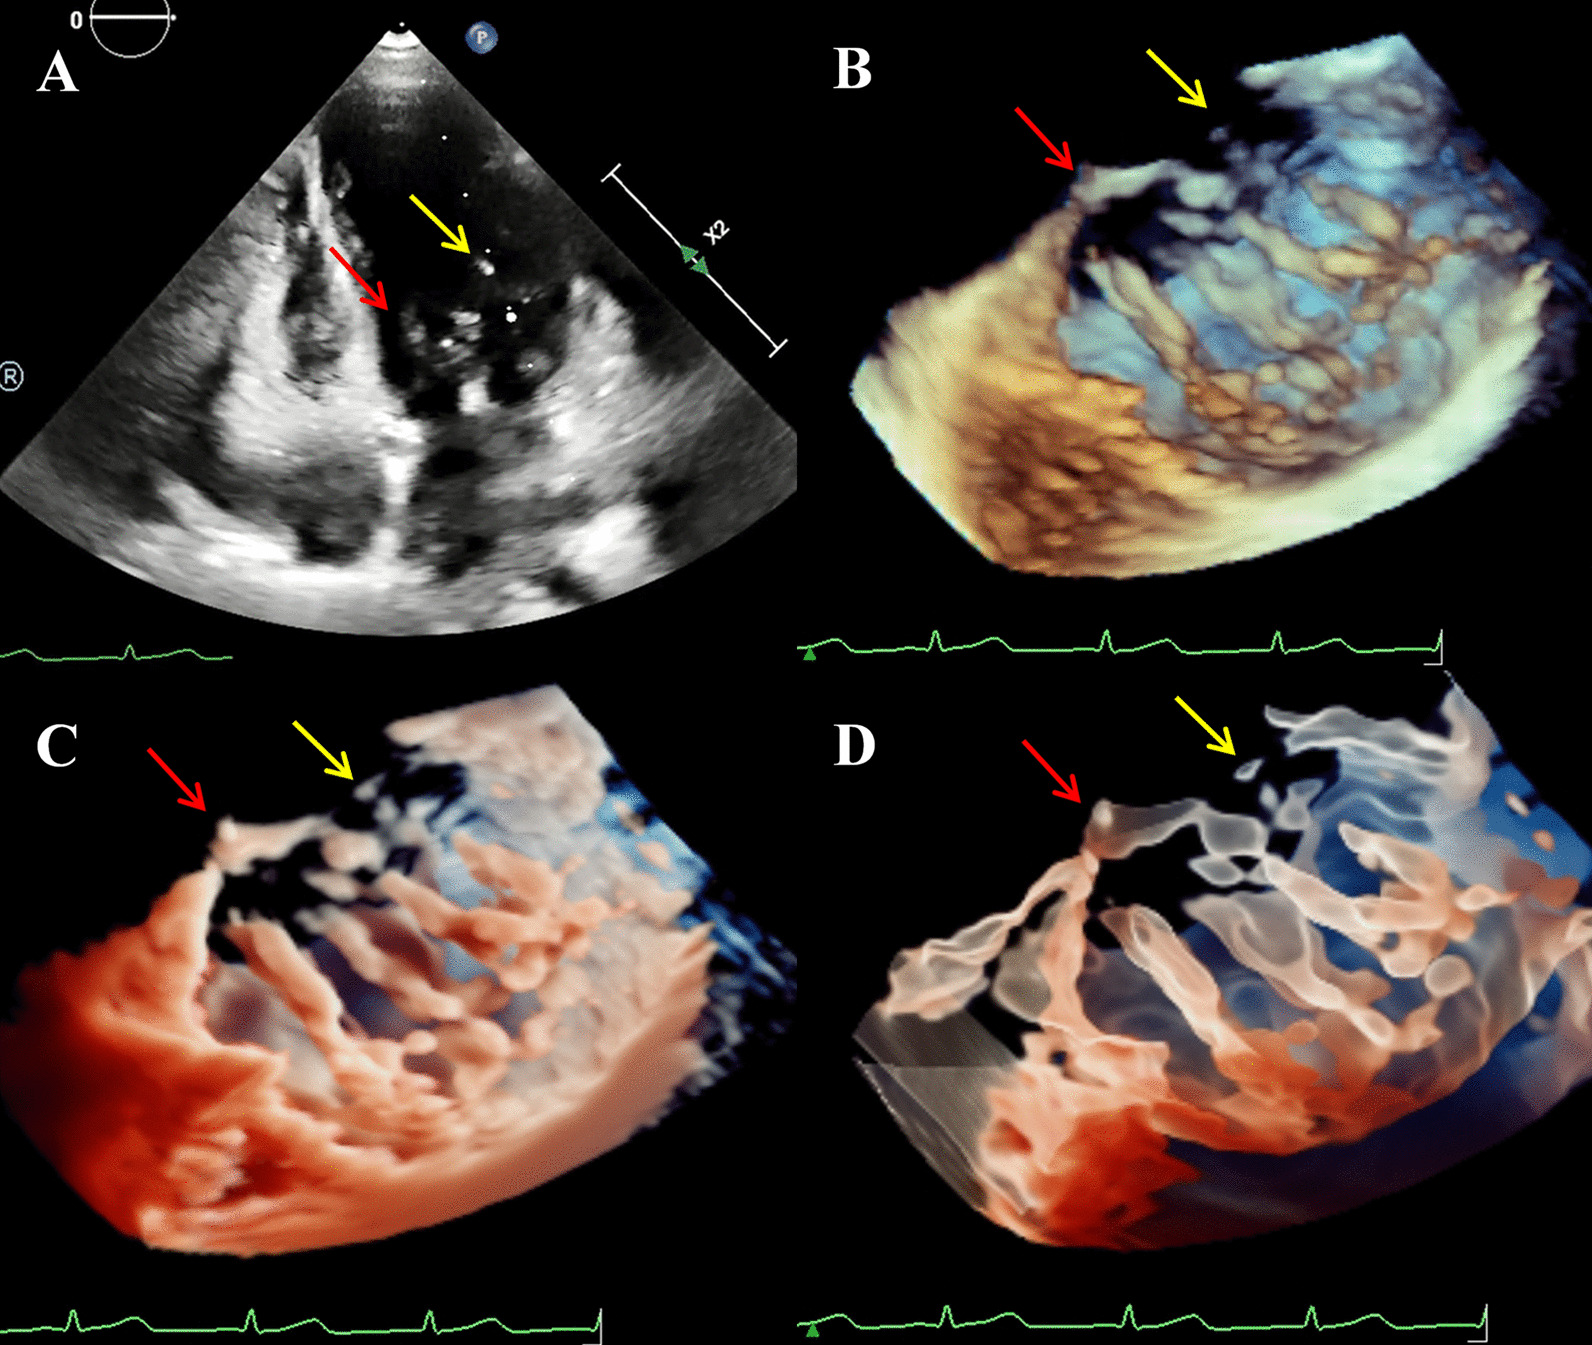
Chordae tendineae. A 2D-TTE showing the long axis of the chordae tendineae. B–D demonstrate the spatial morphological characteristics of the chordae tendineae connecting the two orifices with the four papillary muscles of the mitral valve apparatus by traditional 3DE, TrueVue, and TrueVue Glass. Yellow arrows indicate chordae of orifice 1, red arrows indicate chordae of orifice 2. *2D-TTE* two-dimensional transthoracic echocardiogram, *3DE* three-dimensional echocardiography
